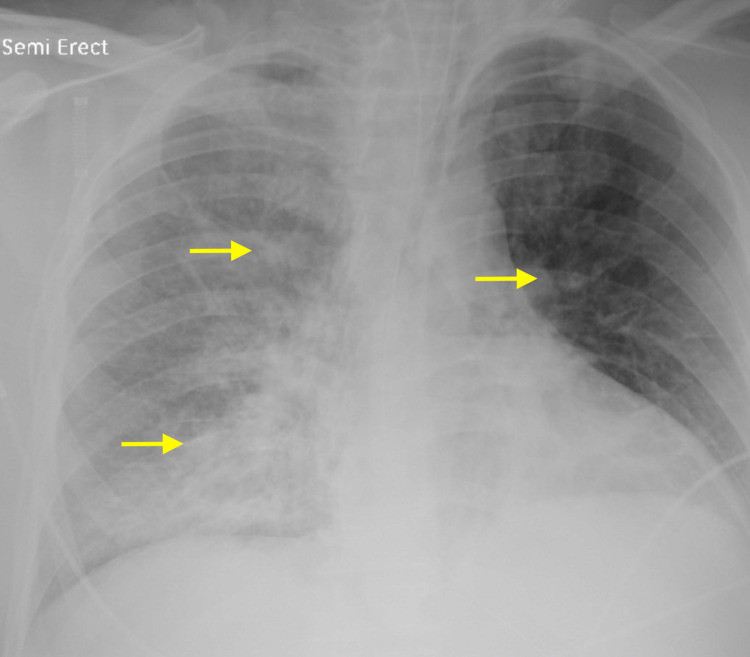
Chest X-ray reveals lung changes characteristic of COVID-19. COVID-19: coronavirus disease 2019
